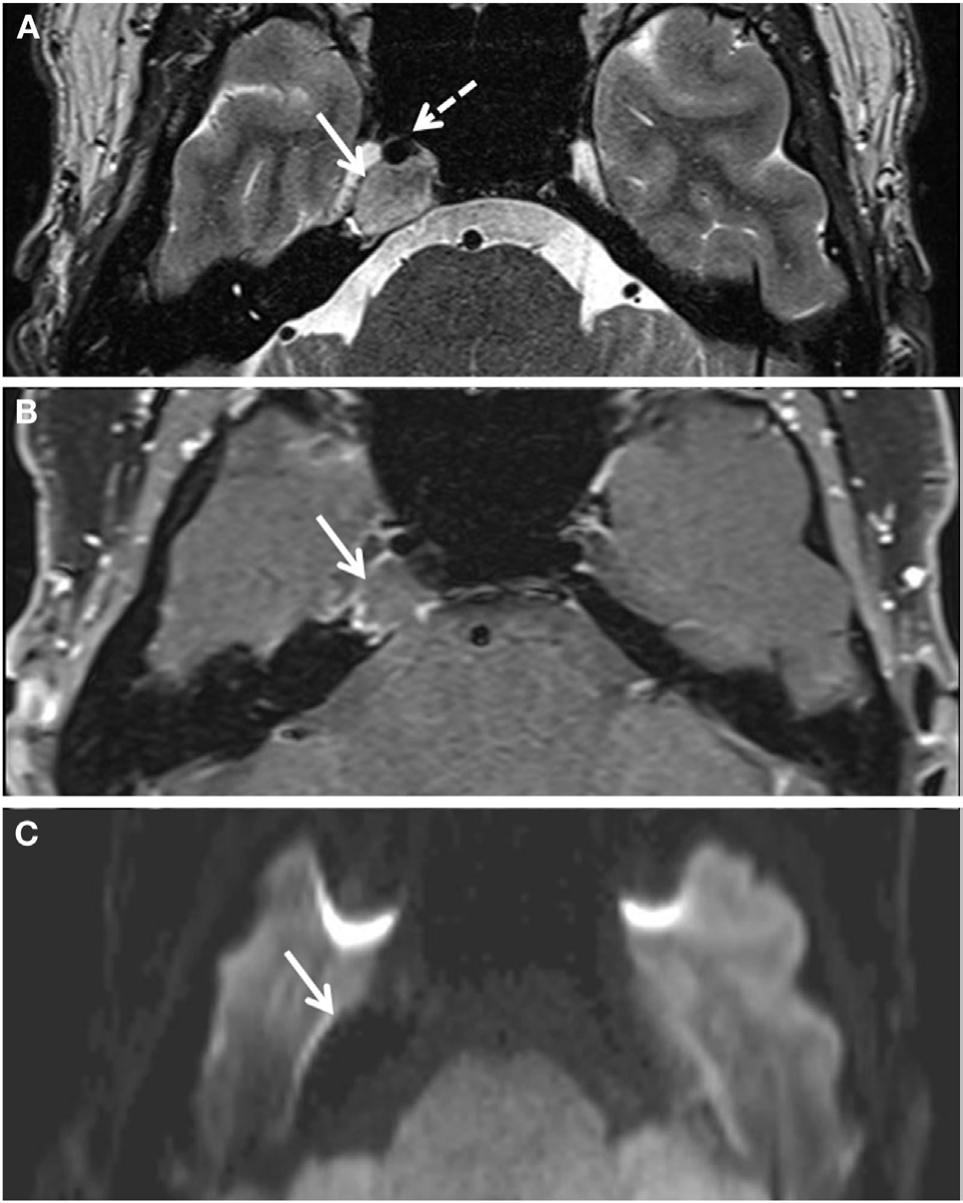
Lesion (white solid arrows) of the right petrous apex as viewed on different axial MRI sequences. (A) T2-weighted imaging shows a slightly heterogeneous lesion with parts isointense to white or gray matter. The dotted arrow shows the internal carotid artery. (B) The lesion is slightly hypointense to brain on T1 VIBE image and demonstrates slight peripheral enhancement following injection of gadolinium contrast. (C) The lesion is hypointense on diffusion trace image.
